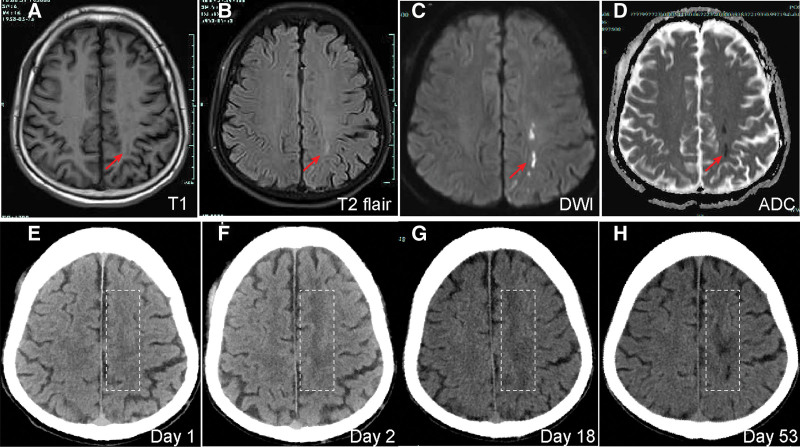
Multimodal imaging and longitudinal evolution of the watershed infarction. (A–D) Admission MRI (day 1): T1-weighted (A) and T2-FLAIR (B) images show subtle changes, while DWI (C) and ADC (D) confirm acute restricted diffusion in the left frontoparietal watershed zone. (E–H) Sequential cranial CT images obtained on day 1 (E), day 2 (F), day 18 (G), and day 53 (H) demonstrate the progression of the lesion from the acute phase to chronic encephalomalacia. The red arrows and the white boxes indicate the lesion in the left parietal lobe and its evolution. ADC = apparent diffusion coefficient, CT = computed tomography, DWI = diffusion-weighted imaging, MRI = magnetic resonance imaging.
